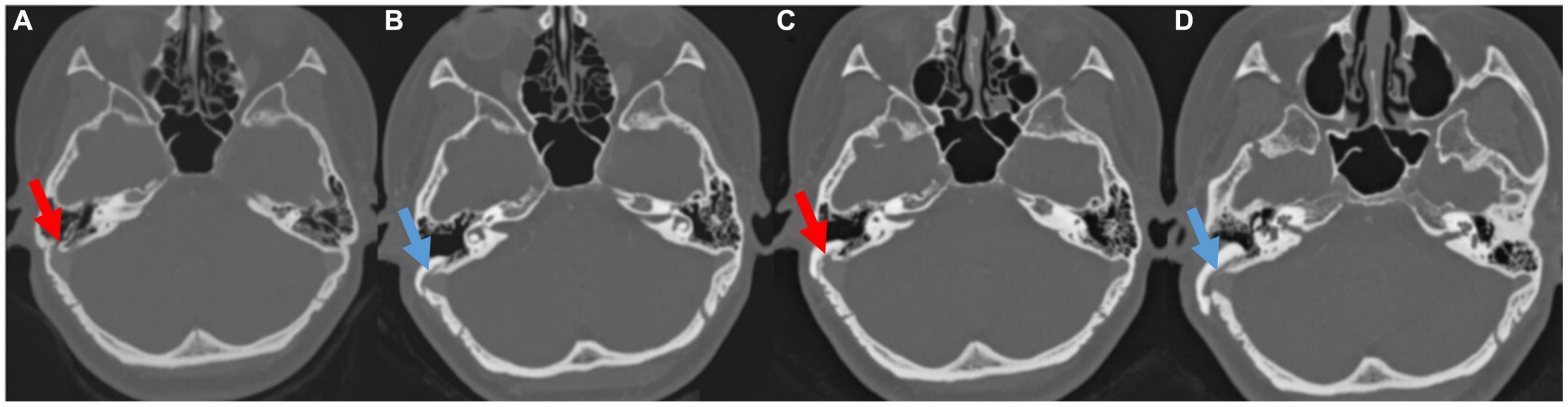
(A,B) Pre-and postoperative temporal bone computed tomography (TBCT) images of Subject 1 showing right sigmoid sinus diverticulum and successful resurfacing after the initial surgery. (C) Pre-revision surgery TBCT images of the same subject showing recurred diverticulum and (D) successfully resurfaced sigmoid sinus after the revision surgery.
